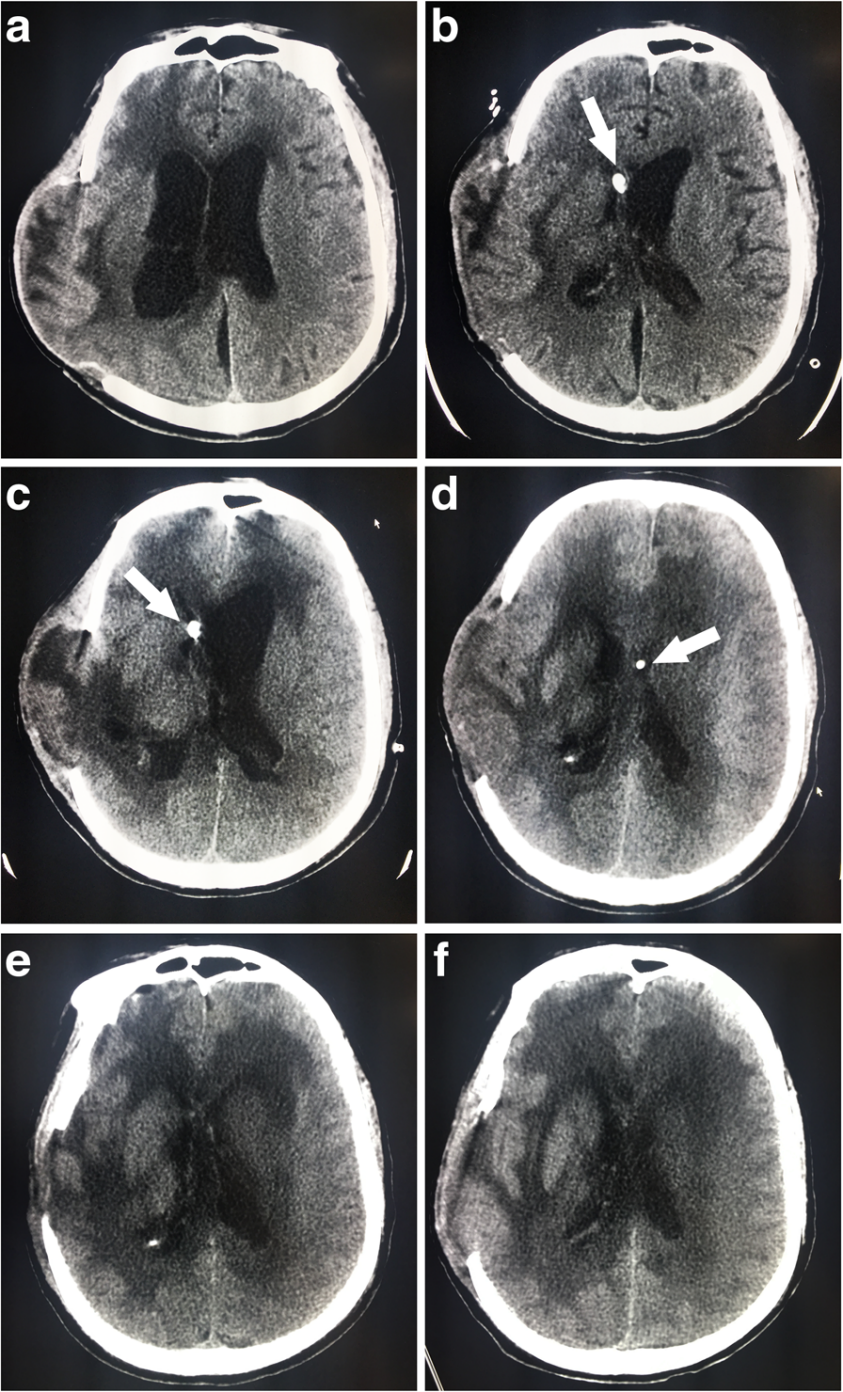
Transient ventricular adhesion due to intraventricular (IVT) polymyxin B administration observed from CT scan. a CT scan obtained on day 1 of the hospitalization, showing bilateral hydrocephalus. b On day 6 of the hospitalization, CT scan showing the contractible right ventricle and enlarged left ventricle after IVT polymyxin B administration through right EVD (arrows). c On day 11 of the hospitalization, CT scan showing the contractible right ventricle and enlarged left ventricle after IVT polymyxin B administration through right EVD (arrows). d On day 18 of the hospitalization, CT scan showing the contractible left ventricle after IVT polymyxin B administration through left EVD (arrows). e CT scan obtained on day 20 of the hospitalization. f CT scan obtained on day 23 of the hospitalization
